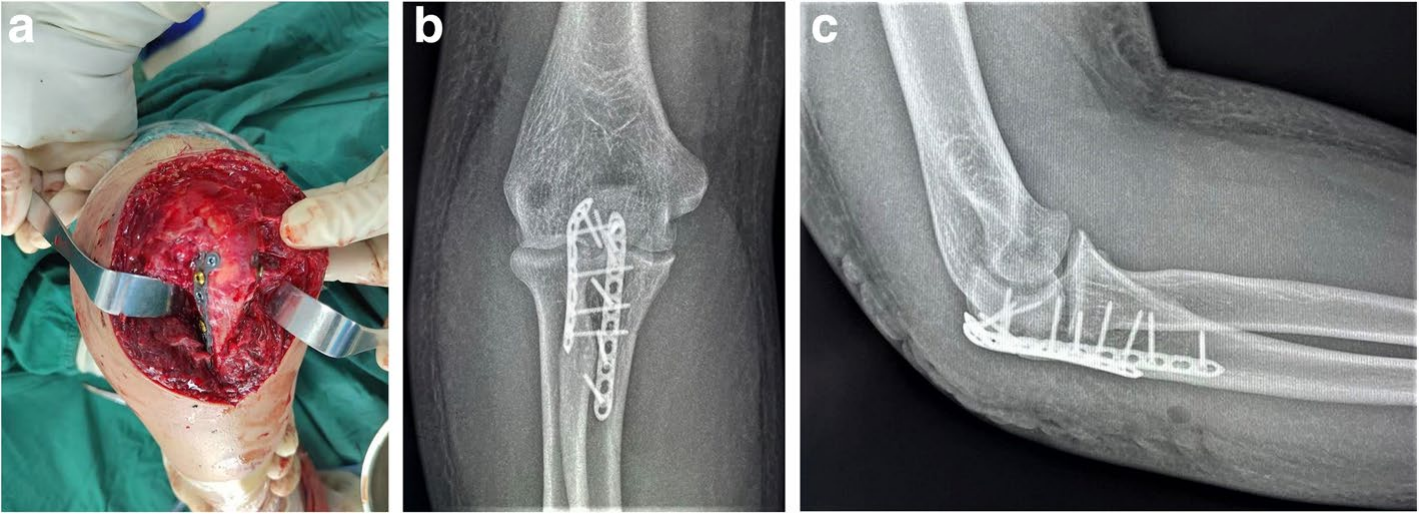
a: Place plates on the posterior medial and posterior lateral sides of the olecranon; b-c: anteroposterior and lateral X-ray film of the elbow joint
